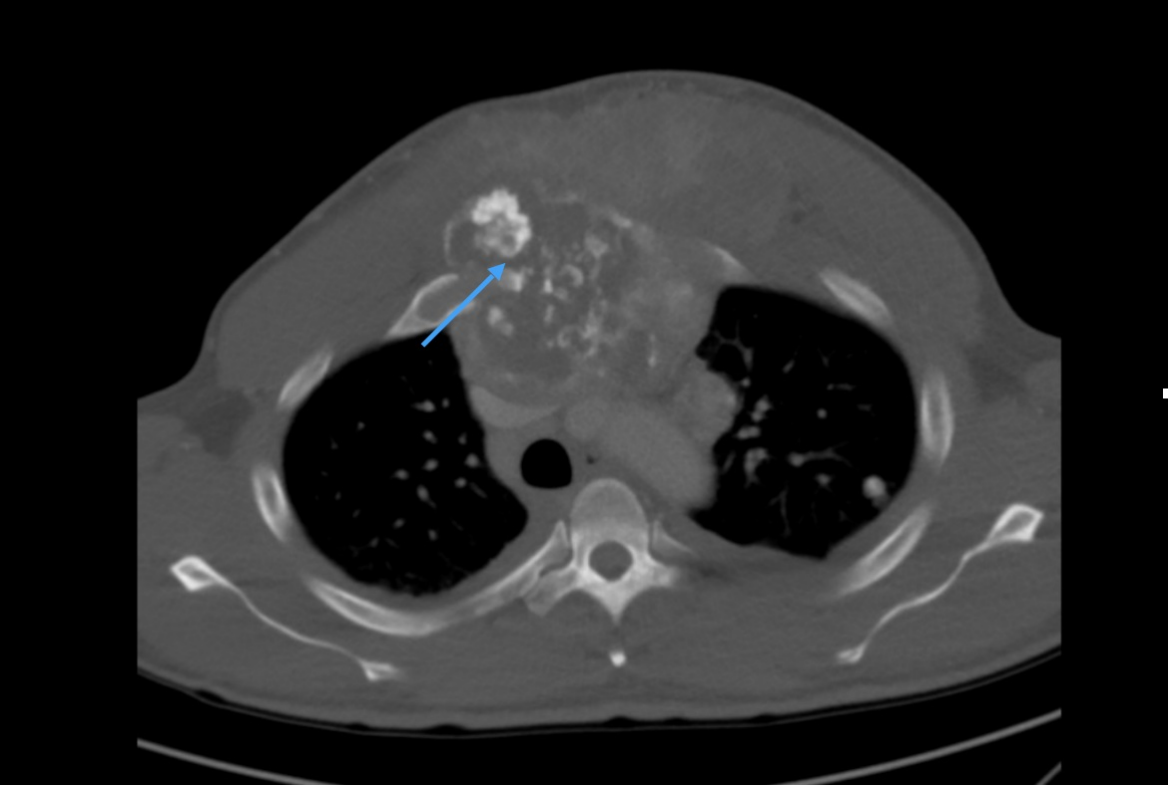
Computed tomography (CT) of the chest with contrast revealing a 11.5 x 15.9 x 11.9 cm anterior calcified mediastinal mass (blue arrow) involving the manubrium and sternum, extending anteriorly into the pectoral muscles
